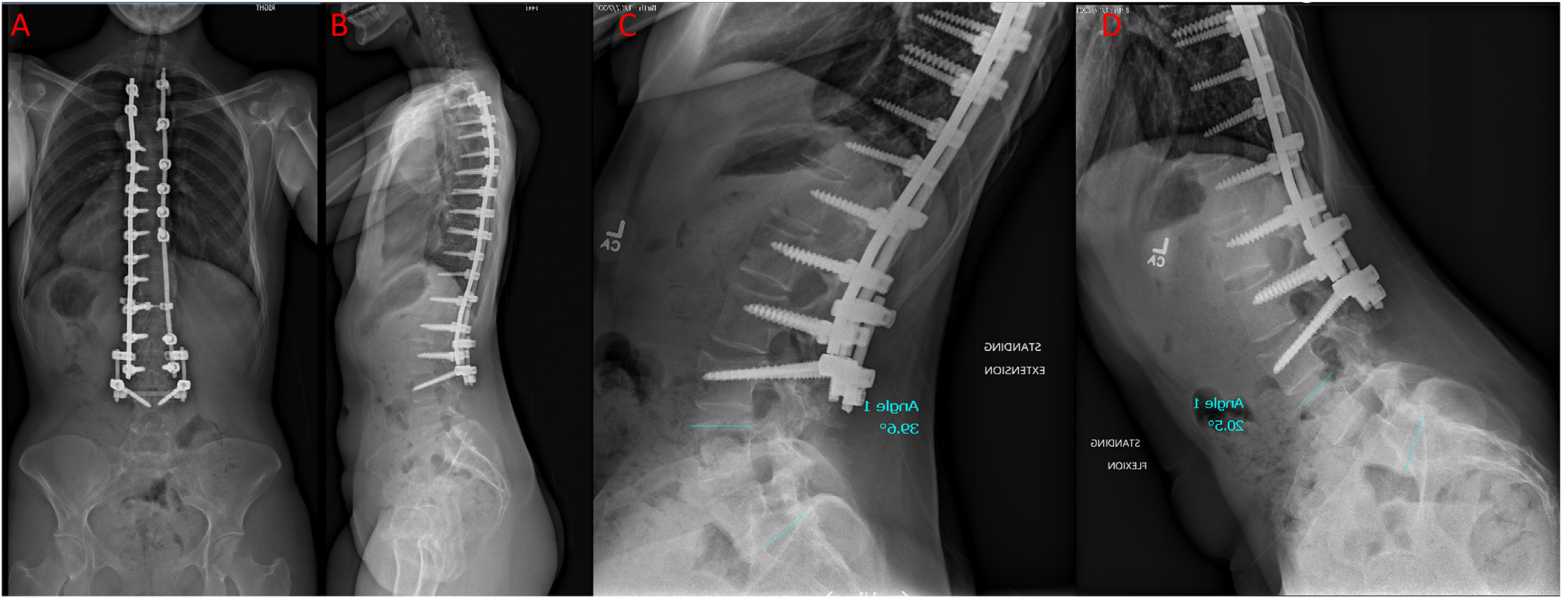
(A–B) Anteroposterior and lateral plain radiograph film after removal of the posterior instrumentation and (C–D) dynamic radiographs showing restoration of motion at L4–S1.
